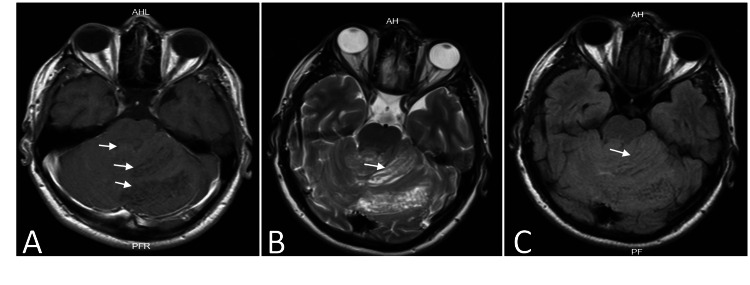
Preoperative MRI. (A) T1-weighted imaging (T1WI) with gadolinium showing an iso- and hypointense left cerebellar hemisphere and vermian lesion without contrast enhancement (arrows) compressing the fourth ventricle. (B) T2-weighted imaging (T2WI) of the lesion with alternating iso- and hyperintense bands related to a tiger-striped sign (white arrow). (C) In fluid-attenuated inversion recovery (FLAIR), the bands are seen as iso and hypointense (white arrow).
